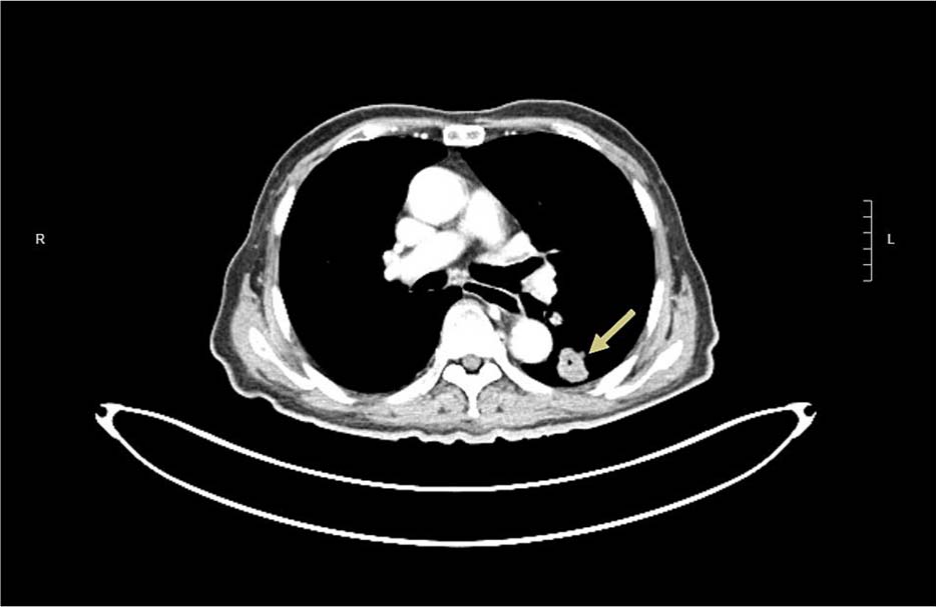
CT revealed a left lower lobe lung lesion (arrow).
